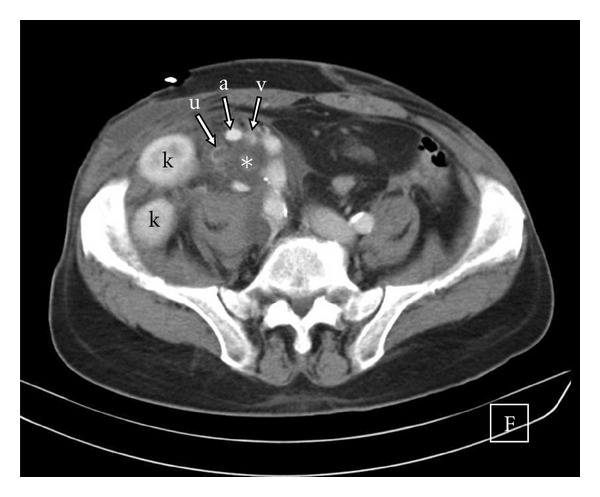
CT scan showing the relationship among the transplanted kidneys (k), the lymphocele (*), the renal artery (a) and vein (v), and the dilated ureter (u).
